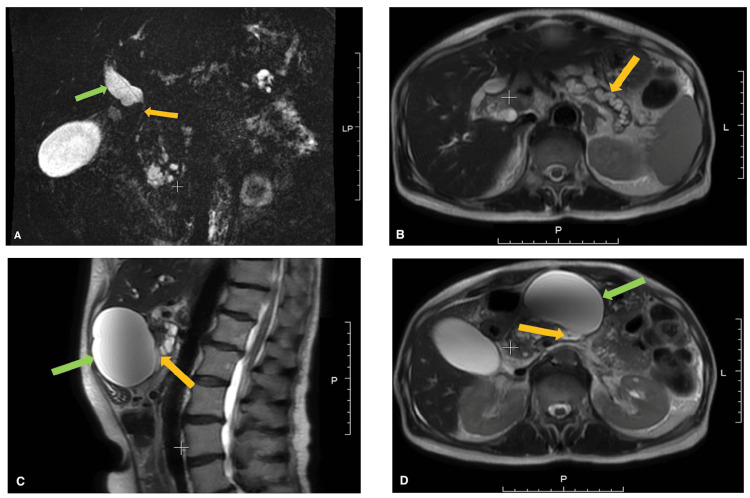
Magnetic resonance imaging findings A) MRCP (Magnetic Resonance Cholangiopancreatography): tubular bile duct stricture 35 mm in length (yellow arrow) with biliary hypertension, diameter of the common bile duct up to 15 mm (green arrow); B) MRI, axial view. Arrow marks a dilated main pancreatic duct (MPD) with a diameter of 12 mm; C) MRI, sagittal view. Green arrow shows pancreatic cyst in the body of pancreas with its communication (yellow arrow) with the MPD; C) MRI, axial view. Green arrow shows pancreatic cyst in the body of pancreas with its communication (yellow arrow) with the MPD.
